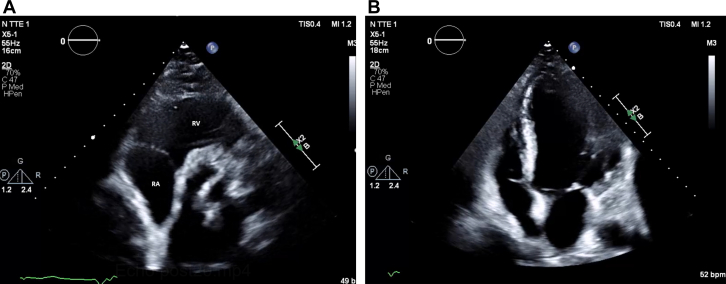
Echocardiography at 3-Month Follow-Up (A) Short-axis parasternal view and (B) apical 4-chamber view revealed complete resolution of vegetation. RA = right atrium; RV = right ventricle.
